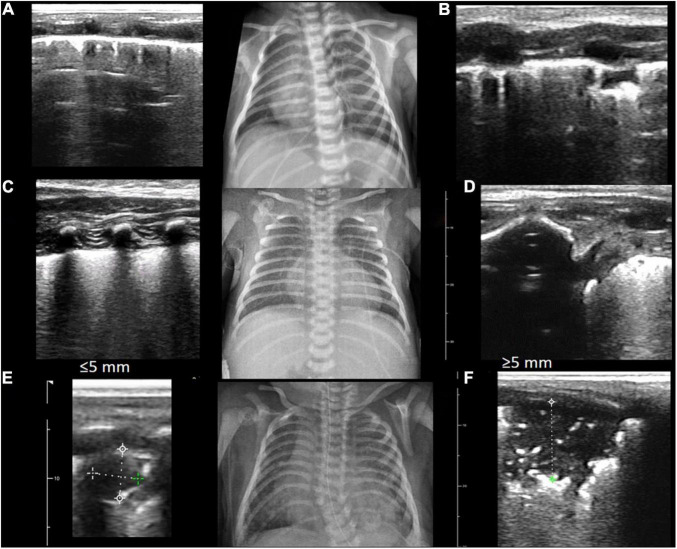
LUS abnormalities and its corresponding radiography are shown. Abnormal patterns found were B-line interstitial pattern 90% (A), irregular/interrupted/thick pleural line 88% (B), compact B-lines 65% (C,D), small consolidations (≤5 mm) 34% (E), and extensive consolidations (≥5 mm) 37% (F).
